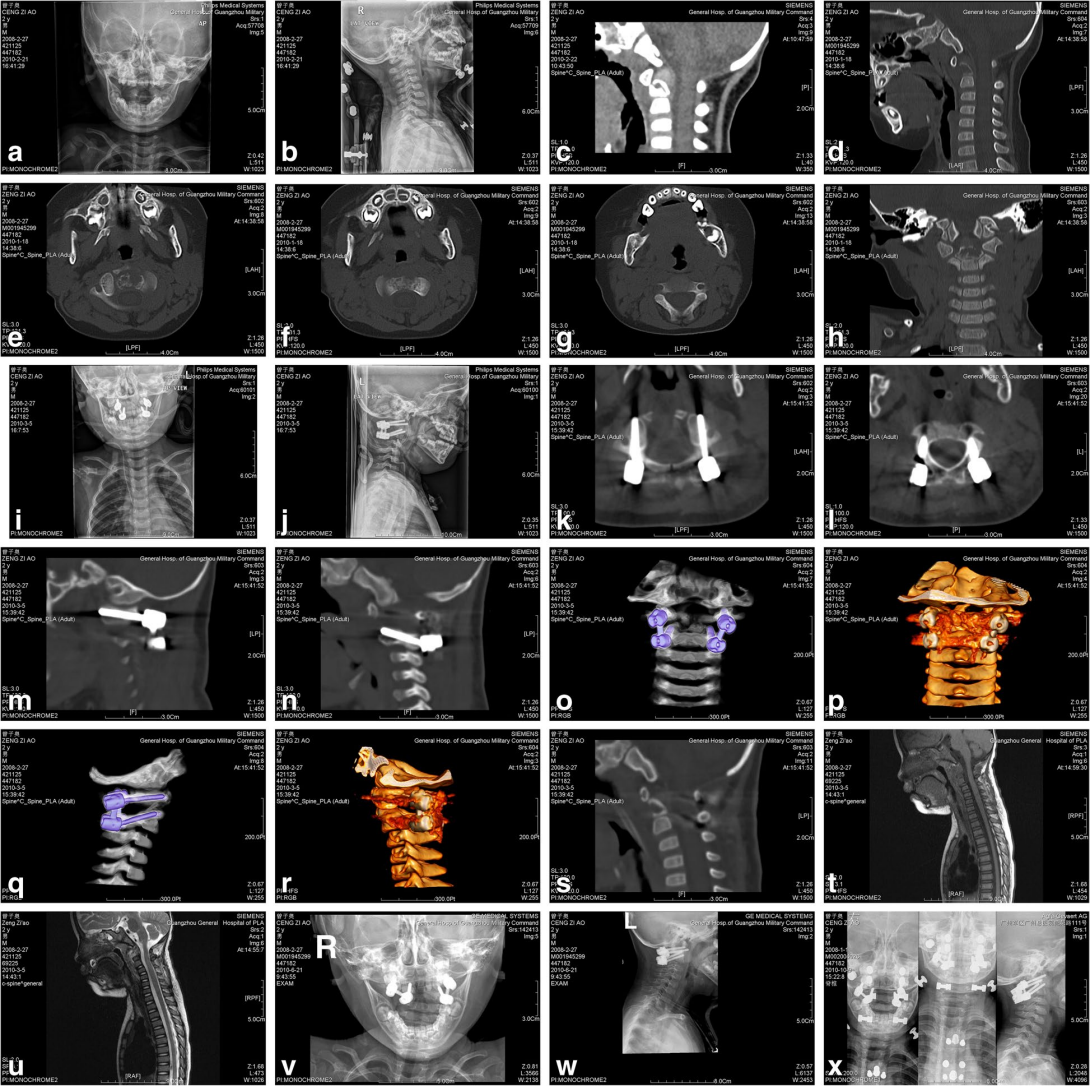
Represents the examination results of a 2-year-old male with eosinophilic granuloma of odontoid process accompanied by atlantoaxial dislocation. He was treated with poterior C1/2 pedicle screw internal fixation followed by transoral lesion resection of odontoid process. The X-rays (see a and b) show anterior atlantoaxial dislocation. As shown in c and d, the CT further reveals atlantoaxial dislocation, bone destruction in odontoid process of C2, and space occupying lesions. According to e, f, g and h, CTs show the lesions are mainly distributed in the odontoid process and lumbar region of C2, and no obvious lesions are found in the basal region. The postoperative X-rays (see i and j) immediately show that the atlantoaxial segment has been completely reducible, and the internal fixation is in good position. As shown in k, l, m, n, o, p, q, r and s, the atlantoaxial segment has been repositioned, and all the screw paths are in good position, without any invasion of vertebral artery holes and spinal canals. The postoperative MRI results (see t and u) demonstrate the odontoid lesion at atlantoaxial level has been removed. According to v and w, X-rays conducted 3 months after operation show that the internal screw fixed in atlantoaxial segment is in place without obvious loosening or displacement. X-rays (see x) conducted 6 months after operation demonstrate that the atlantoaxial internal fixation is firm without any obvious dislocation. In addition, the physiological curve of cervical spine is good, and there is no instability of adjacent segments
